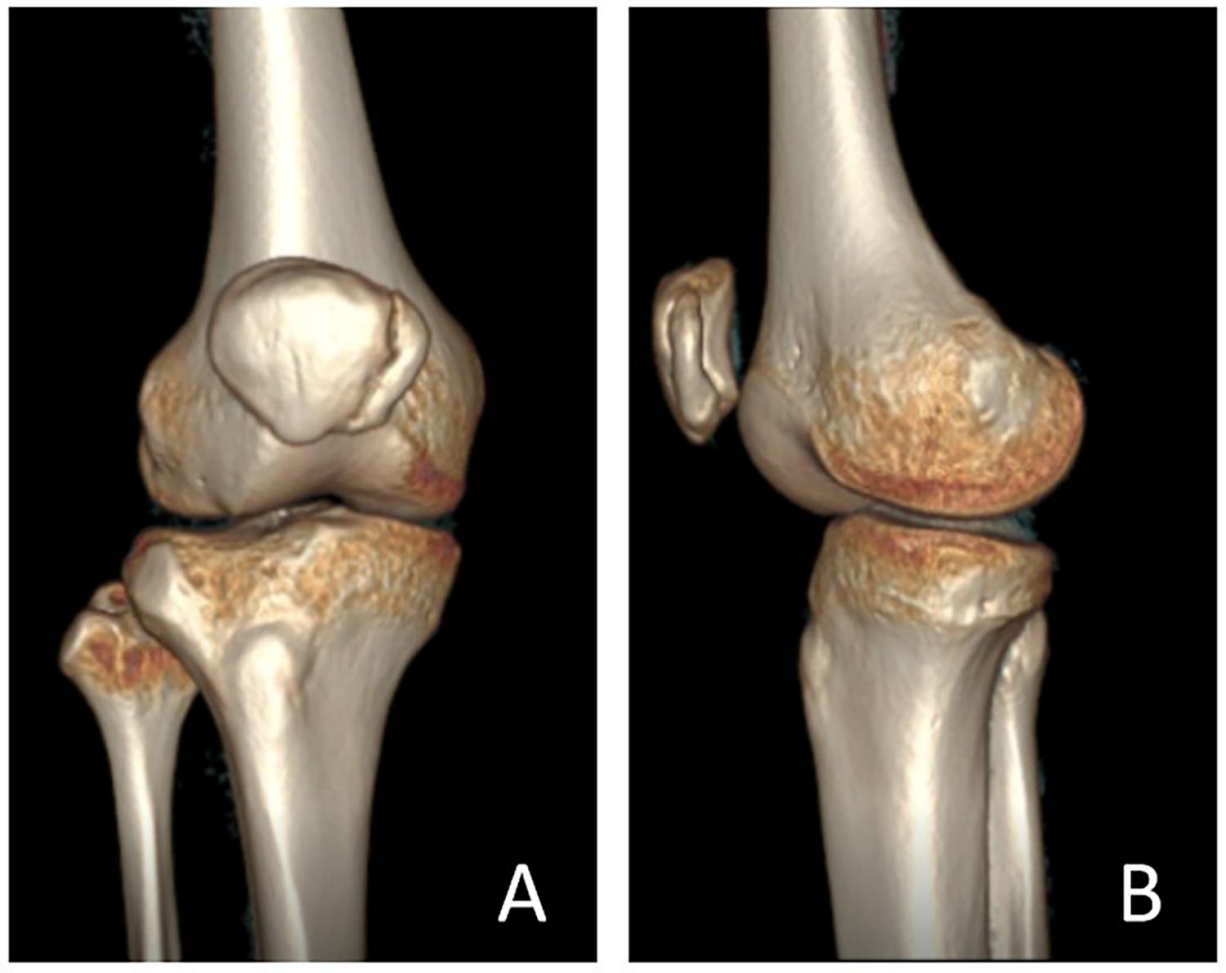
Preoperative right knee CT scan with 3D reconstruction. CT imaging and three-dimensional reconstruction of the right knee demonstrate lateral displacement of the right patella, with a bony high-density lesion located medially. No abnormal bony signal is observed in the proximal tibia, proximal fibula, or distal femur. These findings are consistent with BP of the right knee with patella alta. (A) Frontal view. (B) Lateral view.
